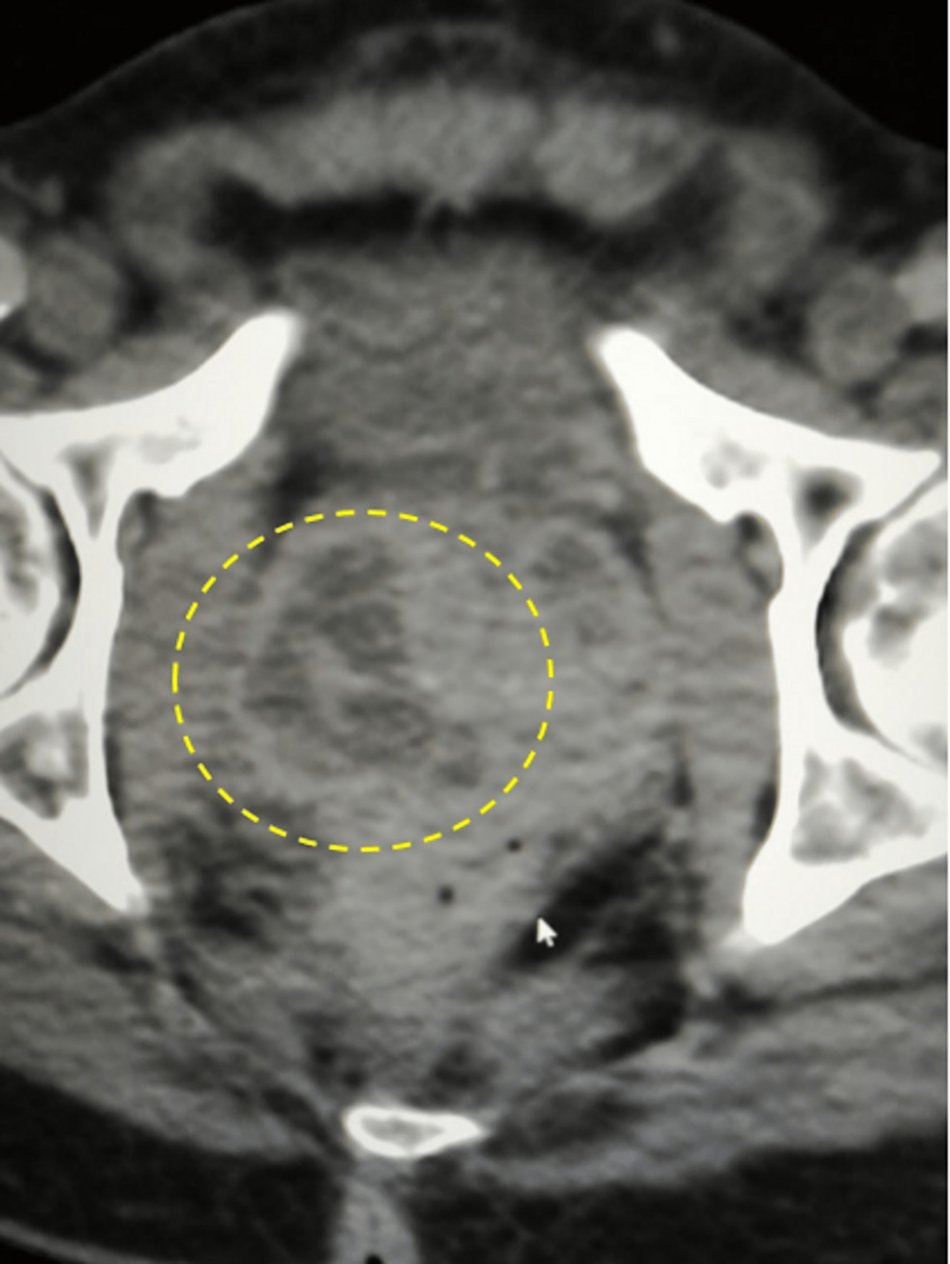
Pelvic CT scan The scan shows loculated prostatic abscesses (dashed circle).
